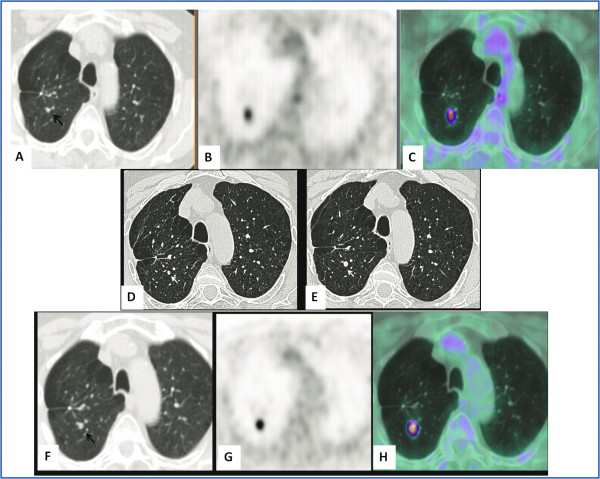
The misinterpreted nodule. ^18^ F-FDG PET-CT (June 2009): CT (a), PET (b), and fused (c) axial images. Focal ^18^ F-FDG uptake is evident in the posterior segment of the upper right lobe of the lung, projecting onto a vascular bifurcation of peripheral lung vessels (black arrow). (d) Axial high-resolution CT image (July 2009): a rounded image interpreted as a mucoid impaction (white arrow) is evident in the posterior segment of the upper right lobe. (e) Axial high-resolution CT image (October 2009): a 7 mm pulmonary nodule corresponding to the site of ^18^ F -FDG uptake (white arrow) is revealed. ^18^ F-FDG PET-CT (November 2009): CT (f), PET (g) and fused (h) axial images. Clear evidence of a pulmonary nodule (black arrow) characterized by intense metabolic activity in the posterior segment of the upper right lobe.
